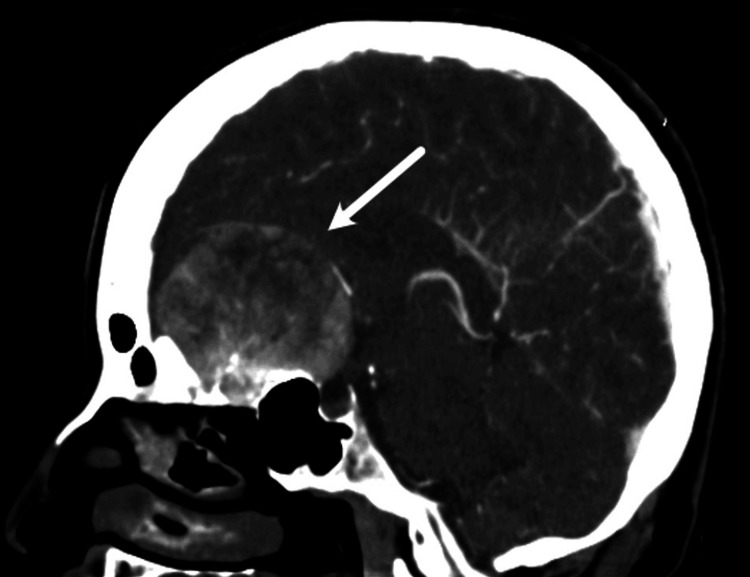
Non-contrast sagittal CT of the brain demonstrates a well-defined, hyperdense suprasellar mass, with significant mass effect on adjacent structures (arrow). CT, computed tomography
